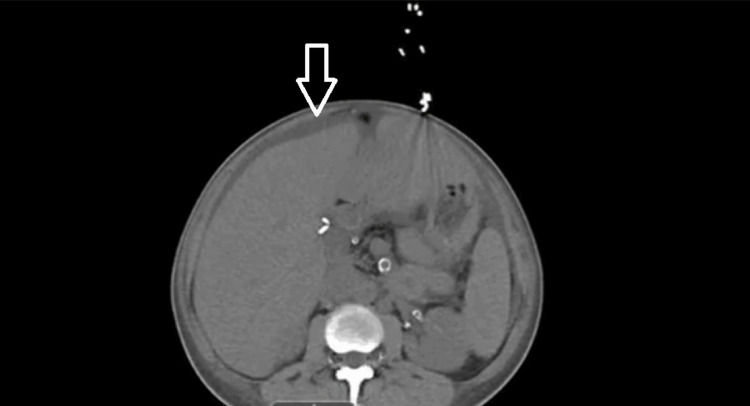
CT of the abdomen and pelvis without contrast (axial view) showing ascites, indicated by the transparent white arrow along the perihepatic region
